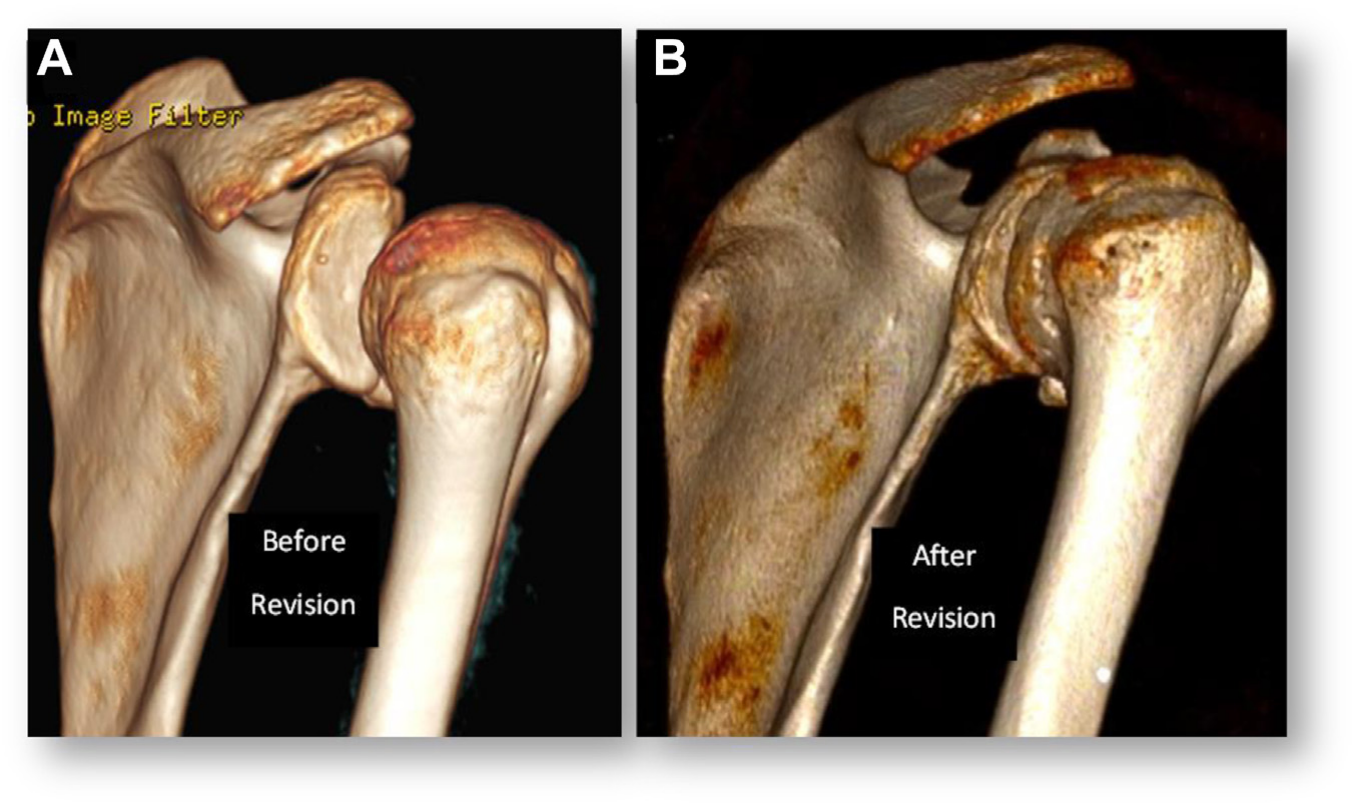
Preoperative and Postoperative 3-dimensional CT scan images showing (A) severe anterior subluxation of the humeral head before surgery and (B) well-centered humeral head after revision with the modified Putti–Platt procedure. *CT*, computed tomography.
